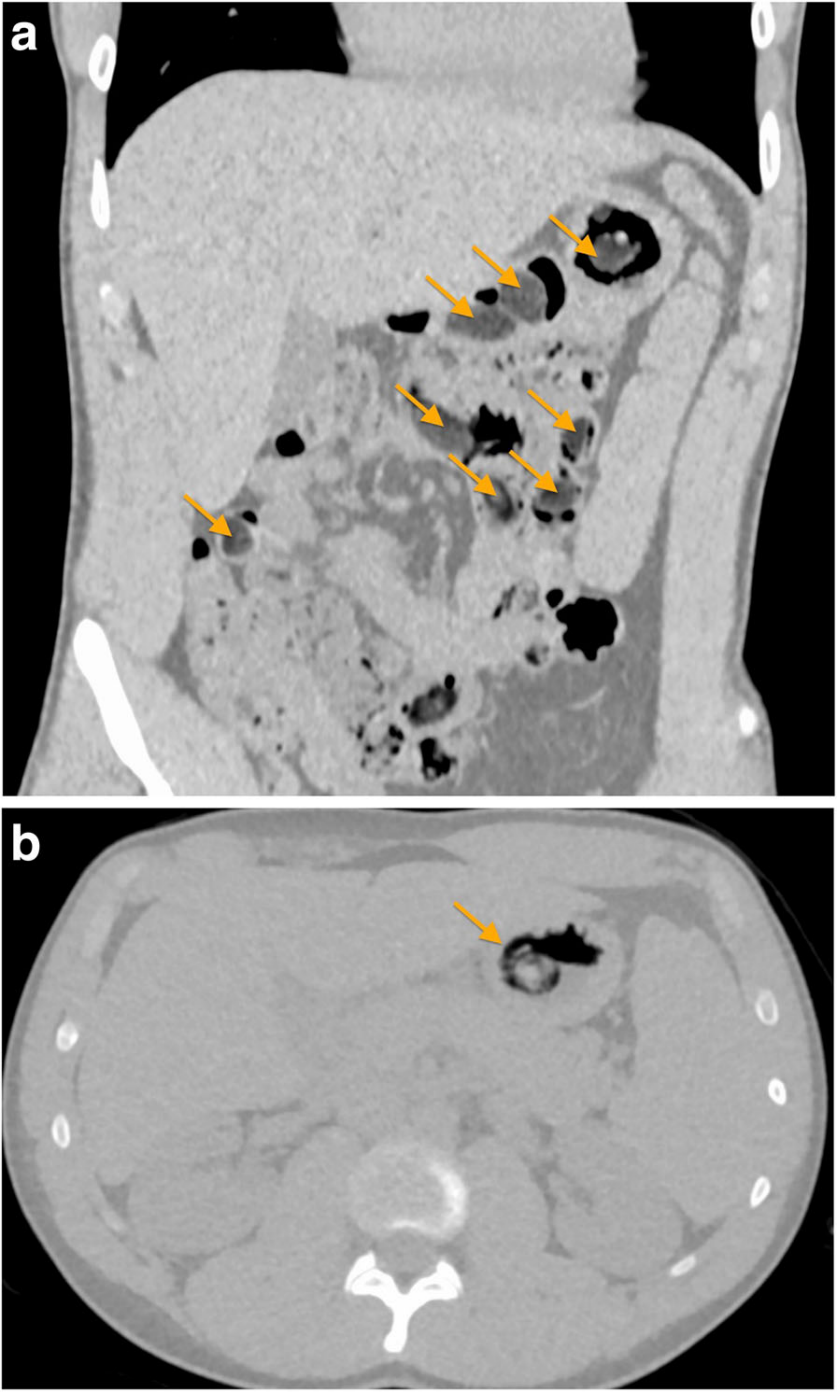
a Multiple intradigestive foreign bodies (*arrows*) situated in the stomach, the duodenum, and small intestine on computed tomography (coronal view). b Single heterogeneous foreign body (*arrow*) containing air measuring 3.1 × 3.2 × 4 cm located in the stomach on computed tomography (axial view)
